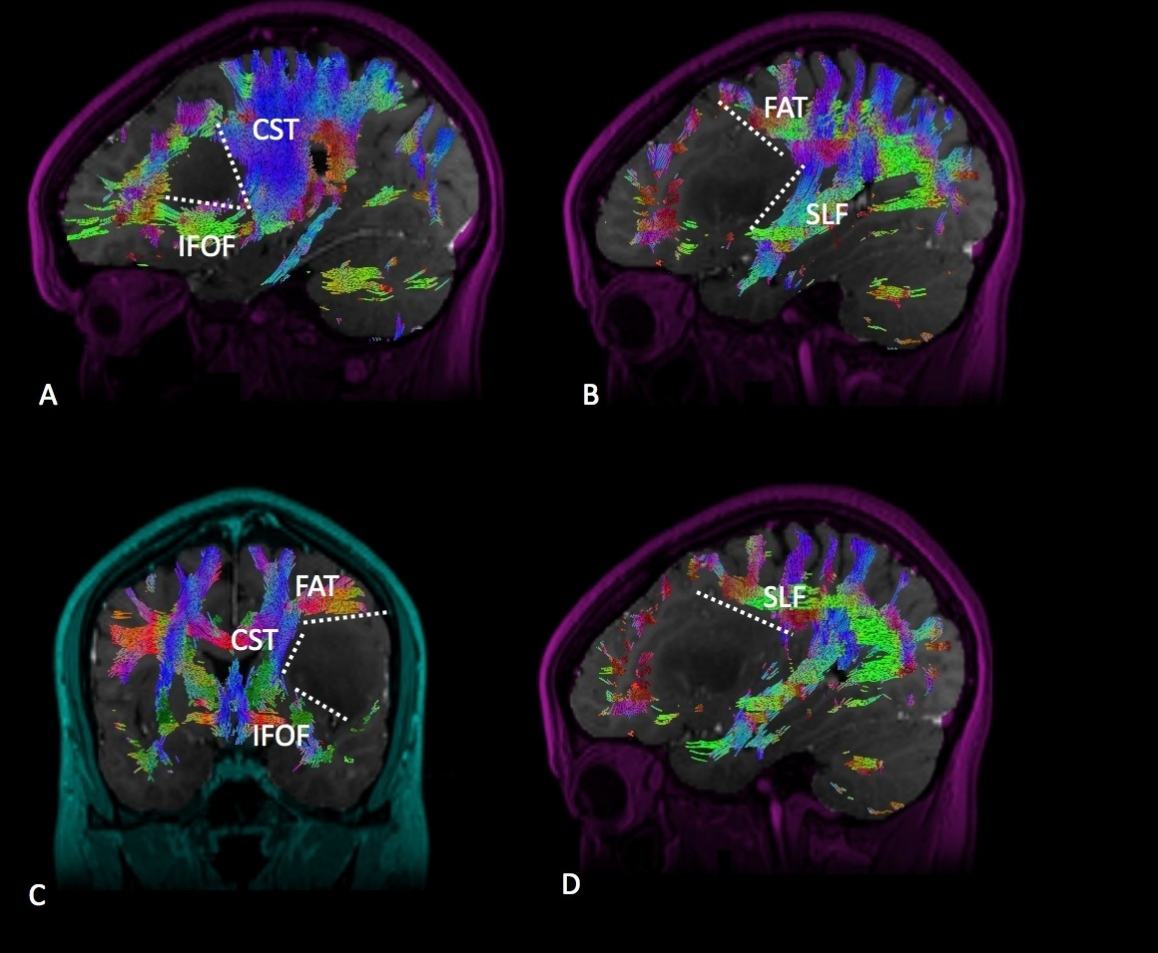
Lateral Posterior Frontal Diffusion tractography enhanced T1 magnetic resonance imaging (MRI) with contrast with (A) sagittal, (B) sagittal, (C) coronal, and (D) sagittal views demonstrating a lateral posterior frontal glioma in relation to the surrounding white matter tracts. Labeled white matter tracts associated are shown as follows: inferior fronto-occipital tract (IFOF), corticospinal tract (CST), superior longitudinal fasciculus (SLF), and frontal aslant tract (FAT). The disconnection pattern is shown as a white dotted line.
